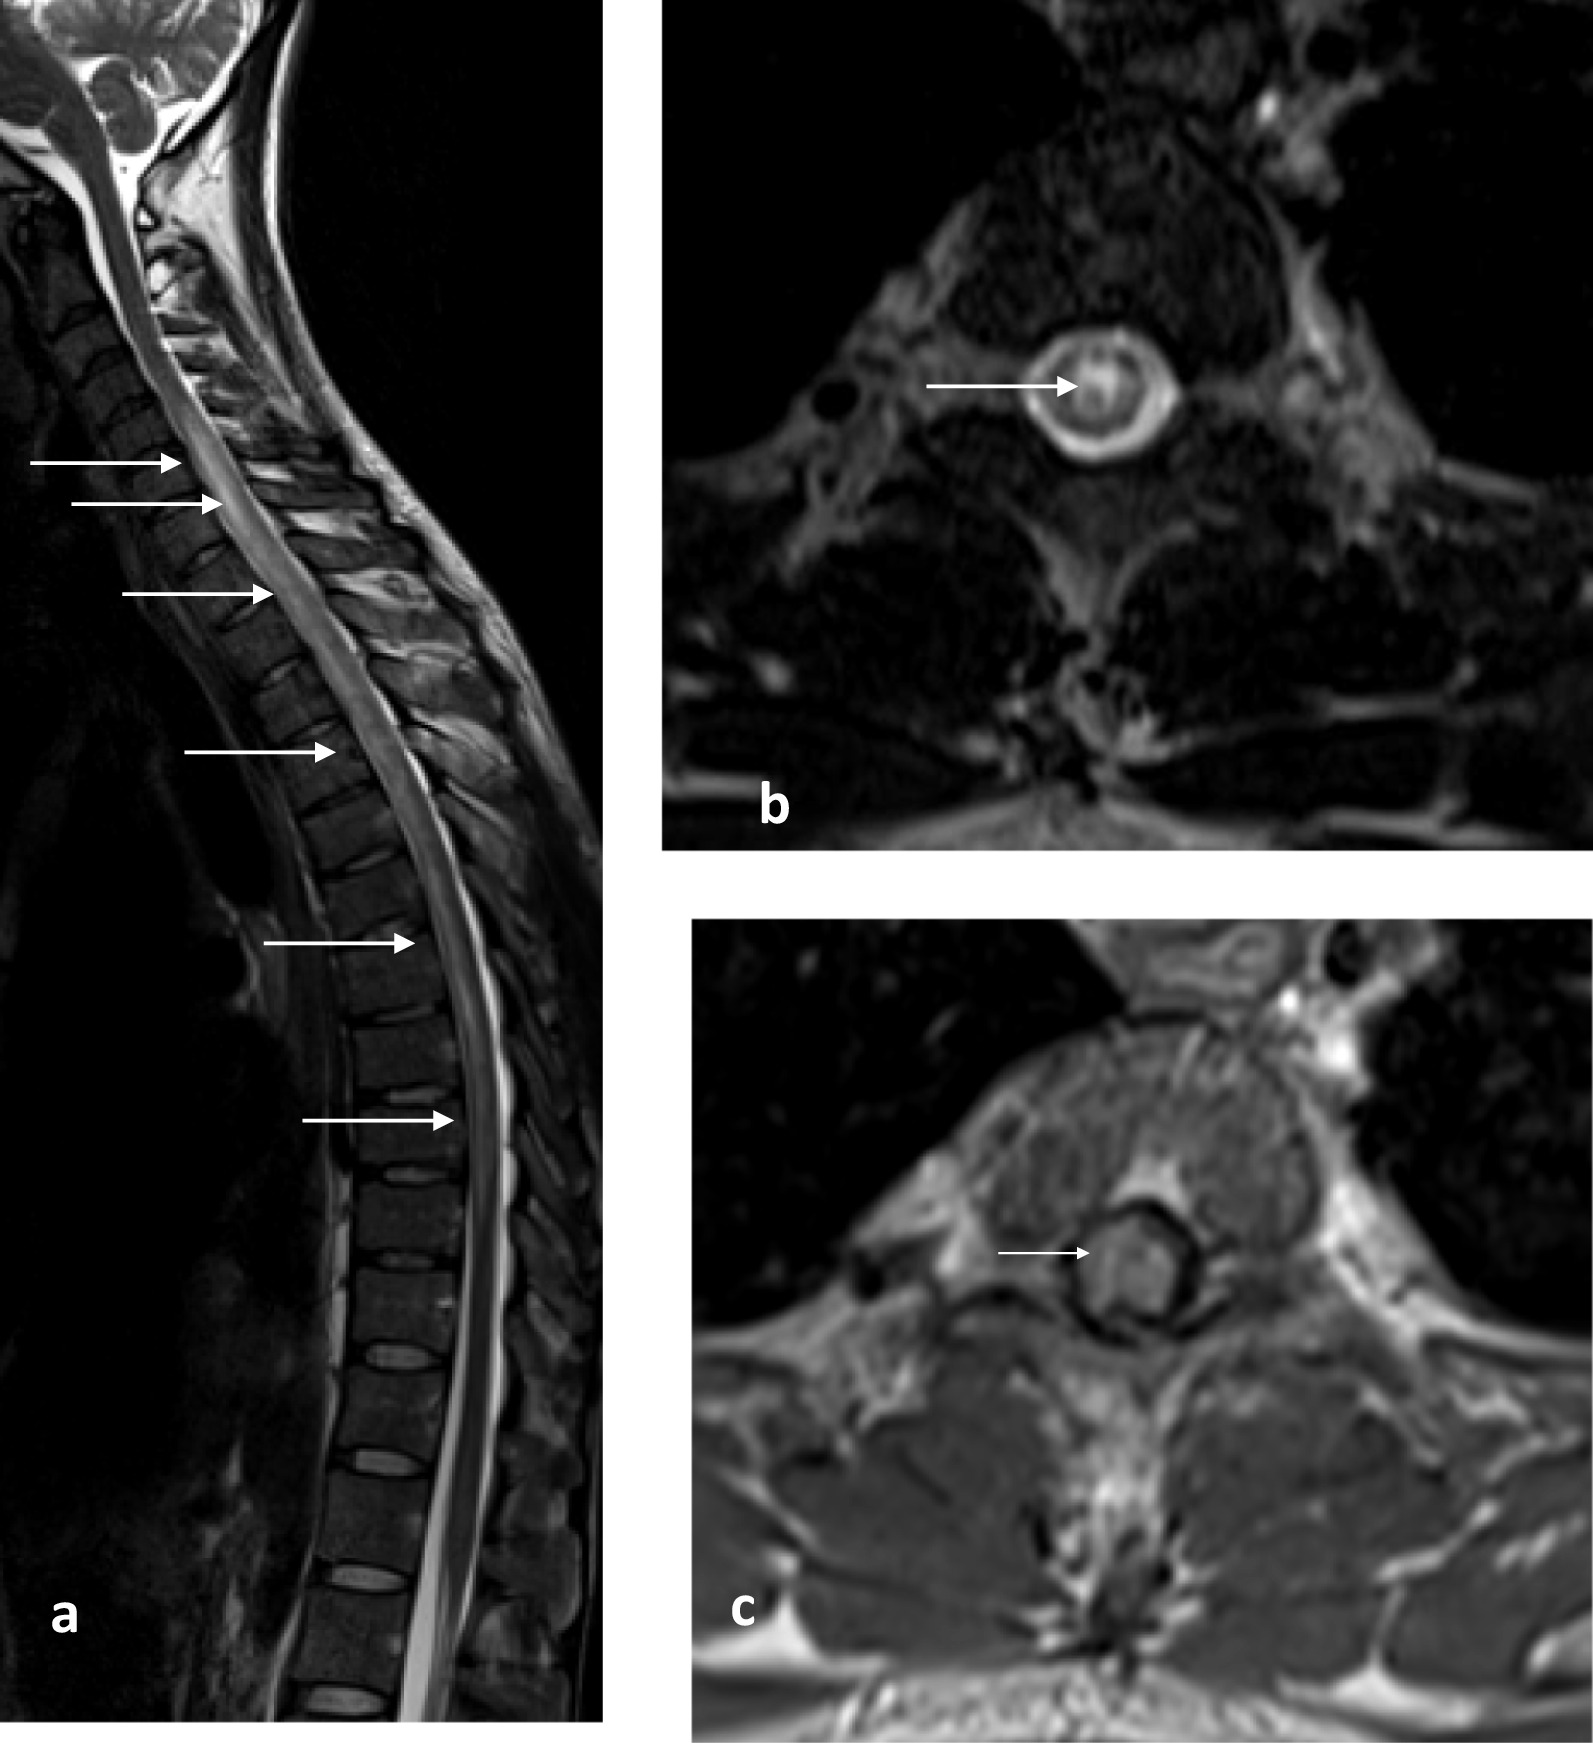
a–c Magnetic resonance images of the spinal cord. a Sagittal T2-weighted image showing longitudinally extensive hyperintensity, extending from C5 to T10 level (arrows); b axial T2-weighted image showing hyperintensity in the cervical cord involving gray matter and appearing as a bright spotty lesion (arrow); c axial T1 post-contrast administration weighted image showing heterogeneous spinal cord enhancement (arrow)
